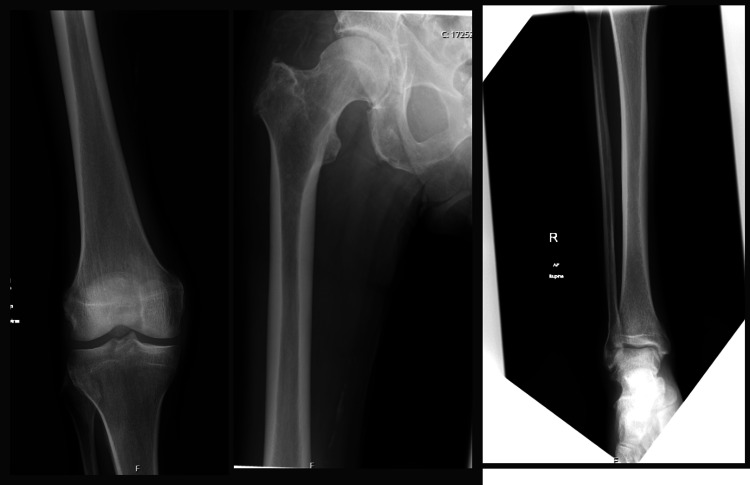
X-ray femur, tibia, and fibula showing mild degenerative changes with no osteolytic lesions.
